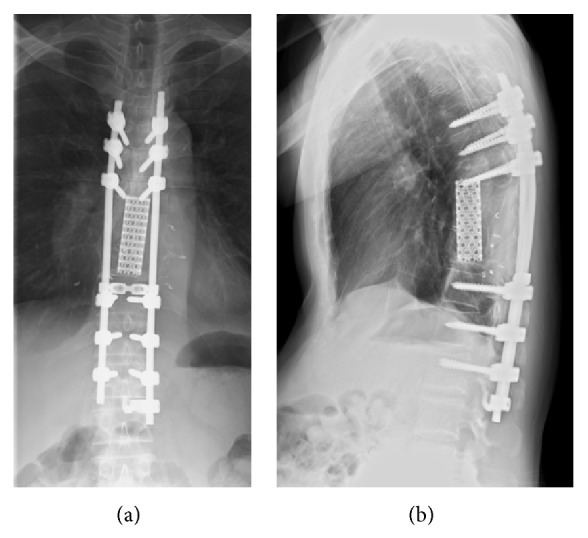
Postoperative radiographs. (a) Anteroposterior, (b) lateral.
